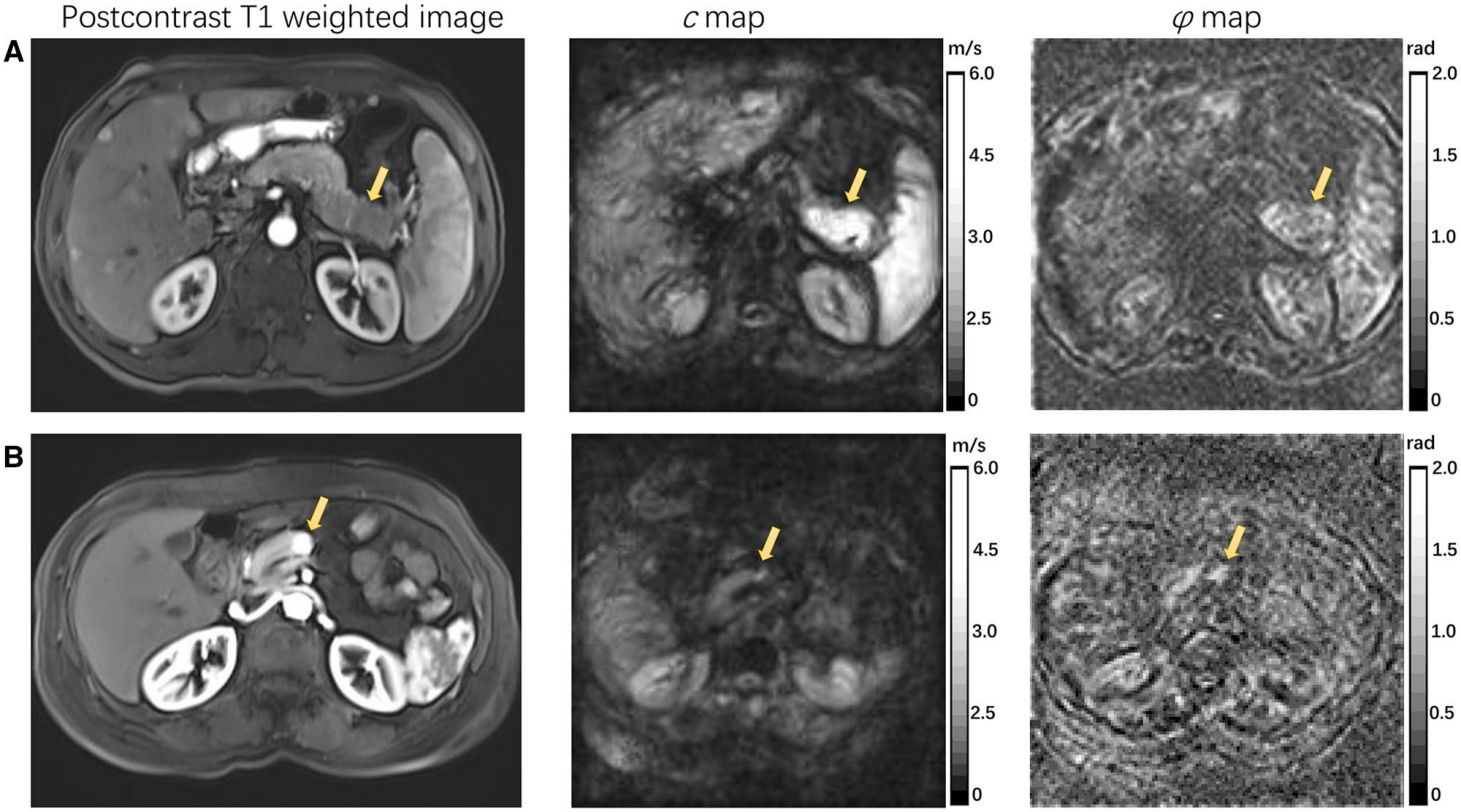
Representative axial T1-weighted images and axial tomoelastography *c* (stiffness) and *φ* (fluidity) maps for two participants. (A) Images in a participant with G3 pNEN (arrows; *c*, 5.92 m/s; *φ*, 1.23 rad). (B) Images in a participant with G1 pNEN (arrows; *c*, 1.85 m/s; *φ*, 1.14 rad).
